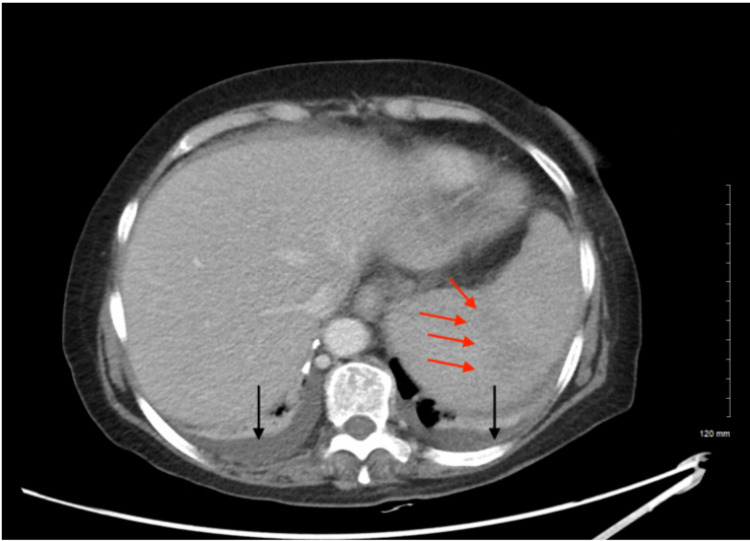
CT of the Abdomen/Pelvis with contrast showing splenomegaly with subtle wedge-shaped low density in the superior spleen (red arrows) concerning for a splenic infarction. Note small bilateral pleural effusions (black arrows).
